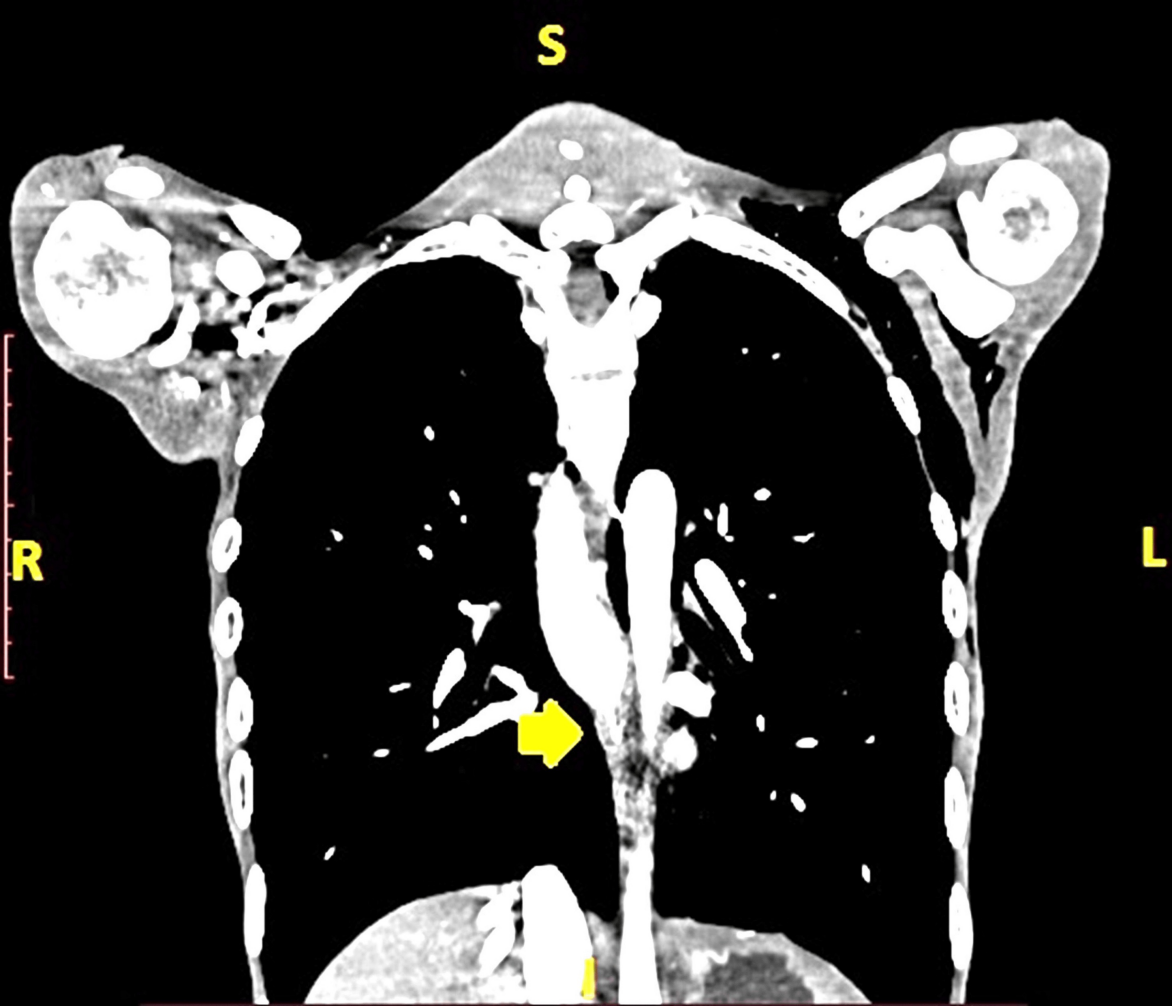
Coronal section CECT scan of the thorax showing lesion involving the middle and lower third of the esophagus with contiguous involvement of the GEJ and completely occluding the lumen (Yellow Arrow). CECT: contrast-enhanced computed tomography; GEJ: gastroesophageal junction
